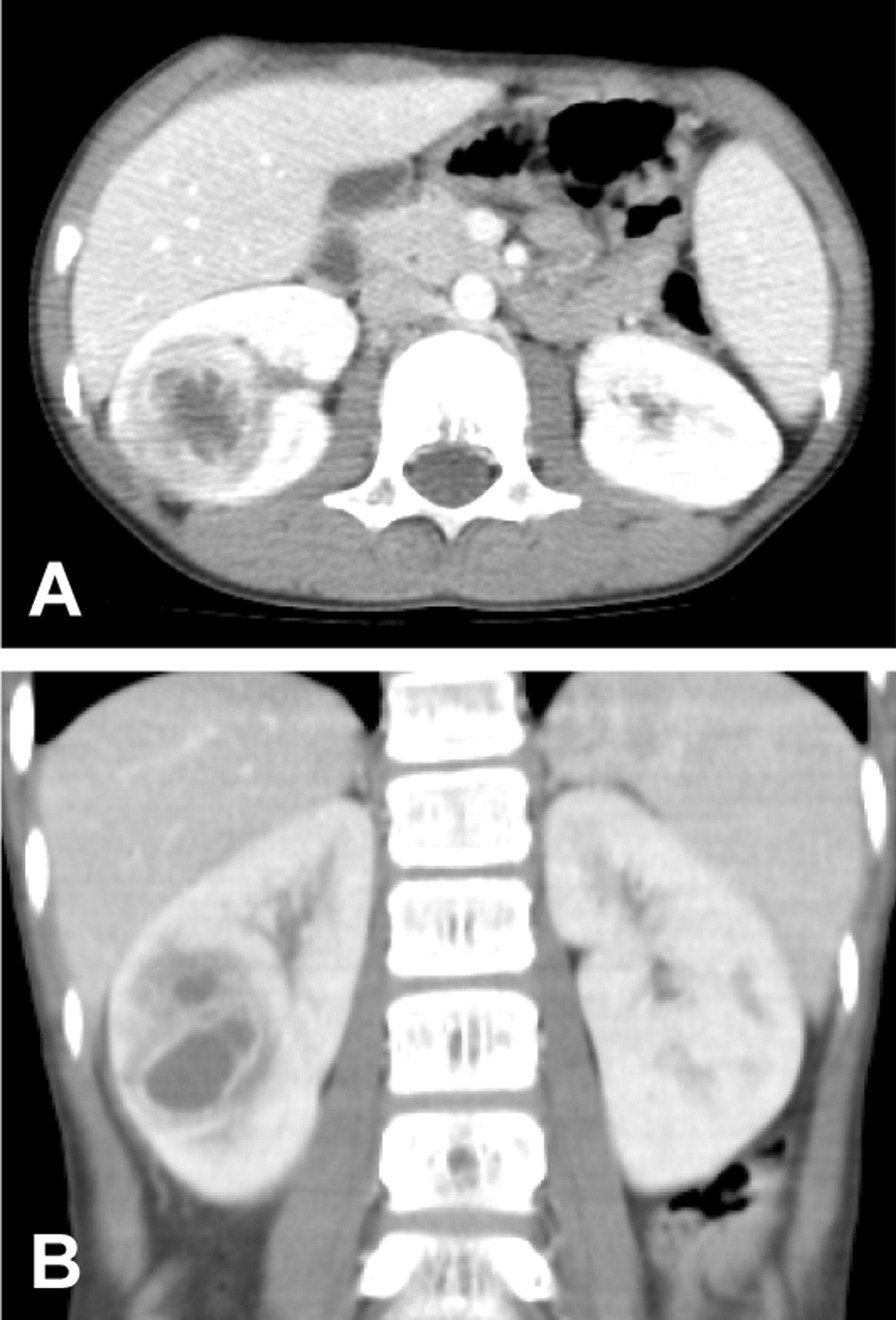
Abdominal contrast-enhanced computed tomography of the patient, A horizontal and B vertical slices
